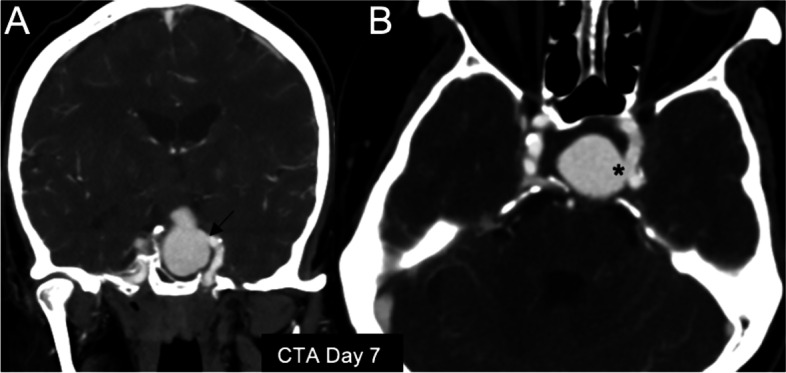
Computed tomography angiography images obtained at our hospital clearly demonstrated an intratumoural pseudoaneurysm originating from the left cavernous internal carotid artery. A Coronal reconstruction image and (B) axial reconstruction image showed an early enhancing pouch directly communicating with the left cavernous internal carotid artery (black arrow in (A), asterisk in (B))
